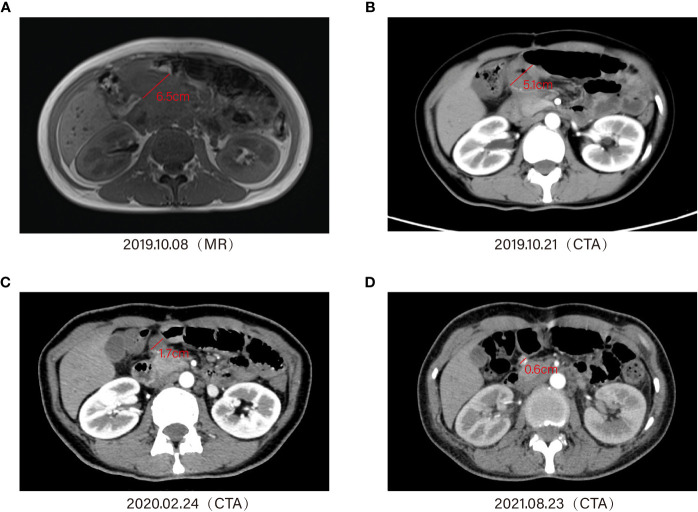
Timeline of treatment and diagnostic assessment about the patient who received a pembrolizumab injection of 100 mg, intravenous drip regularly. Magnetic resonance imaging (MRI) and enhanced computed tomography (CT) showed the lesion shrinking gradually (A–D).
